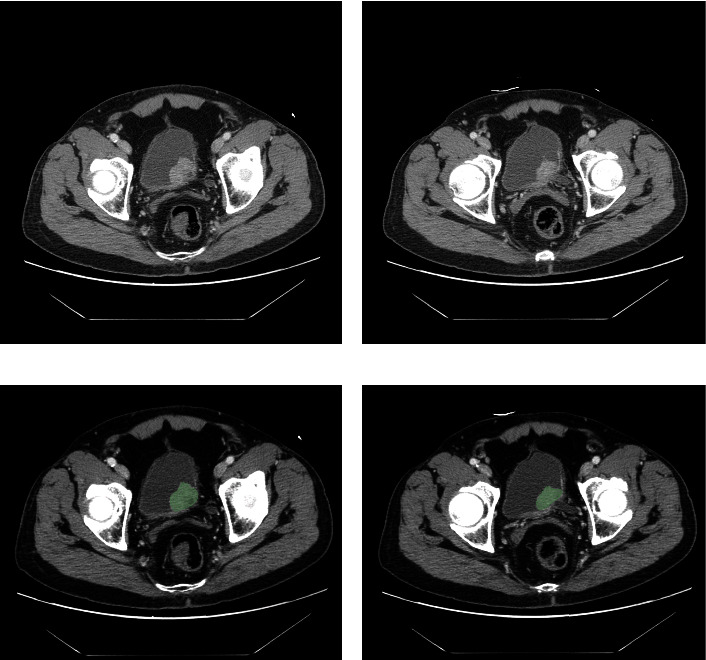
(a-b) The image shows the CT of a 64 years old bladder cancer patients; (c-d) the Identification of ROI in CT image.
